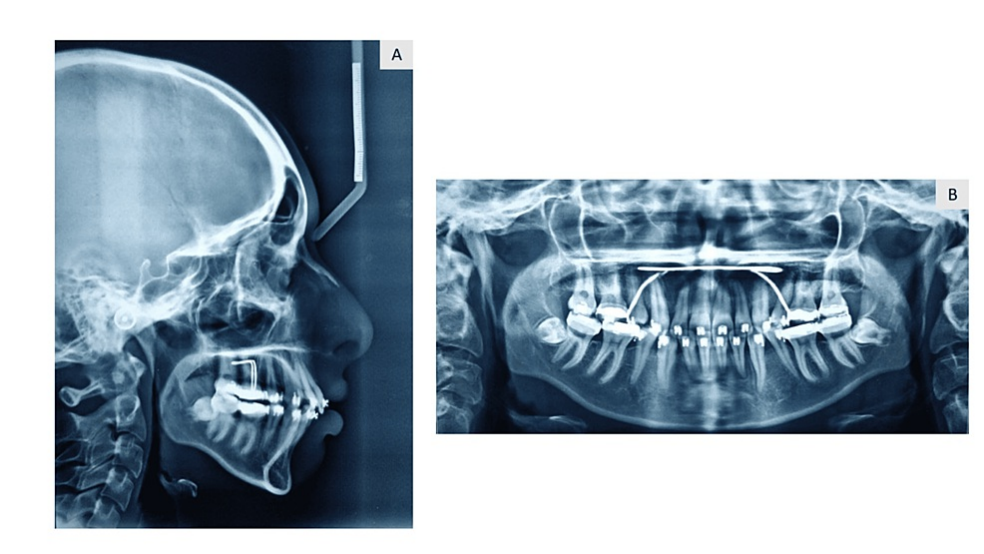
Post-treatment radiographs before settling of occlusion. (A) Lateral cephalogram and (B) OPG (orthopantomogram)
